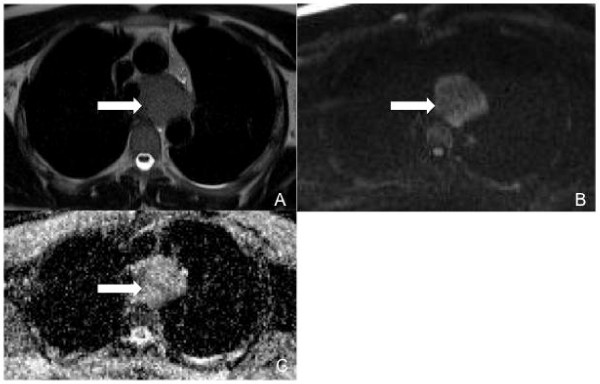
MRI of the patient's mediastinum. (A) T2-weighted half Fourier acquisition dingle shot turbo spin echo (HASTE) sequence on axial plane shows a mediastinal solid mass. (B,C) DWI at b1000 shows a homogeneous high signal intensity and a non-homogeneous low signal intensity on ADC map (white arrows).
